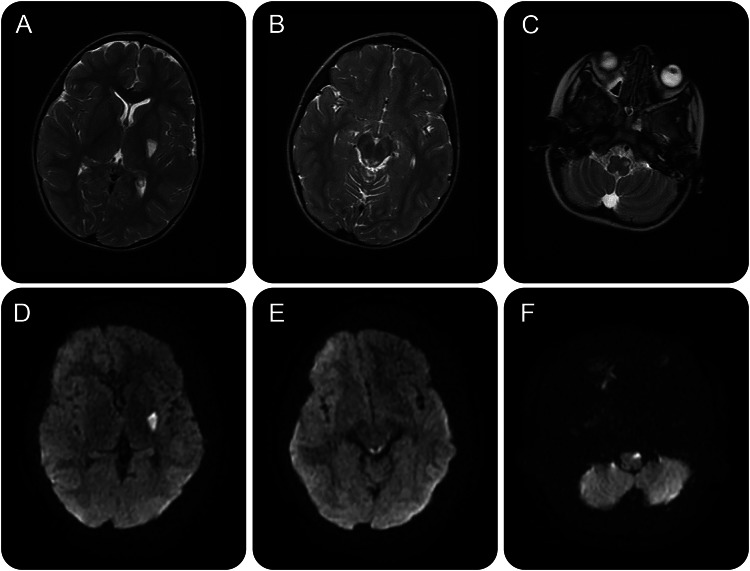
MRI head (age 2 years) Axial T2-weighted images (A–C) reveal hyperintensities involving the left globus pallidus, midbrain, and medulla, with diffusion restriction evident on diffusion-weighted sequences (D–F).
